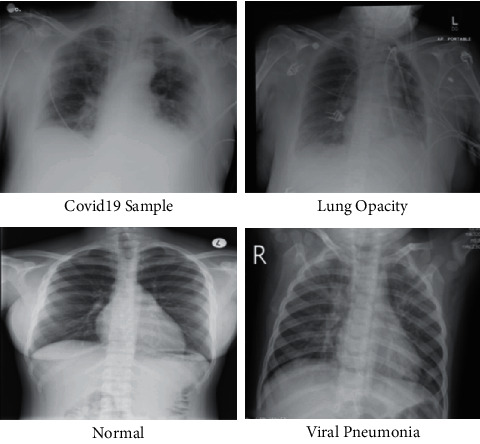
Sample image of COVID-19 Radiography dataset.
